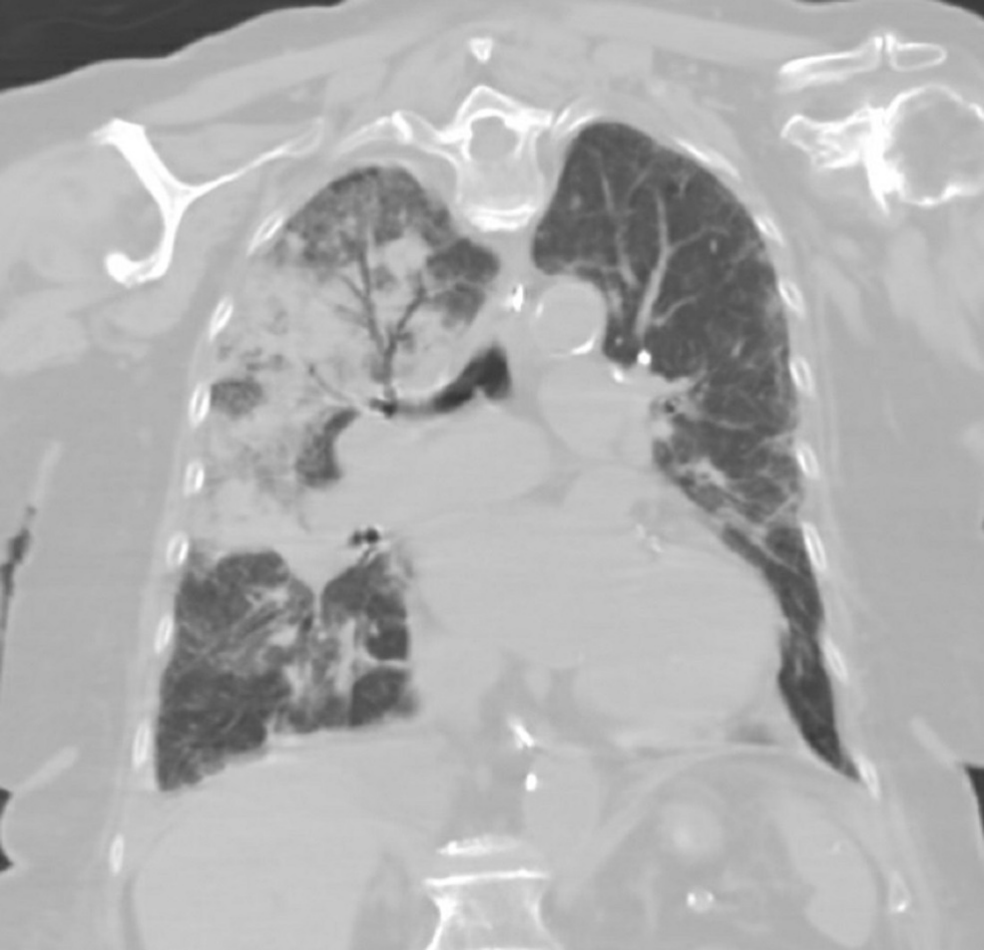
Coronal view of the computed tomography scan of the chest revealed consolidation and air bronchograms involving the right upper lobe.
